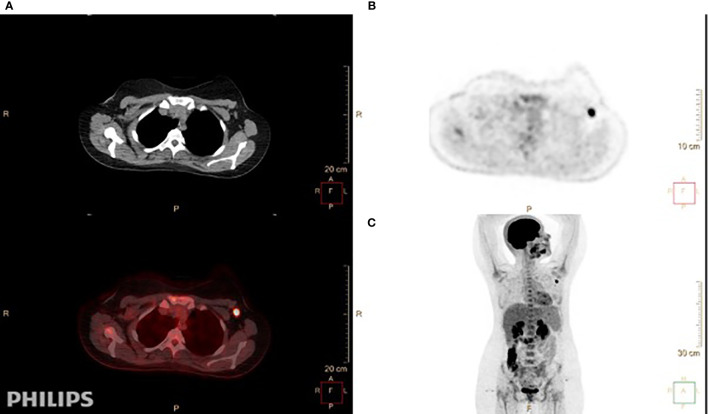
A 24-year-old-female patient with invasive ductal carcinoma in the left breast, grade II, estrogen receptor (ER)-positive, progesterone receptor (PR)-positive, and HER2-negative. She had T1, N1, Mx disease. (A) Axial ^18^F-FDG-PET/CT showed fluorodeoxyglucose (FDG)-avid left axillary lymph nodes. The largest and most avid measures 1 × 1.6 cm with SUV_max_ of 9.8. (B, C) Axial (B) and coronal (C) views demonstrate high FDG uptake for the enlarged node in the left axilla.
